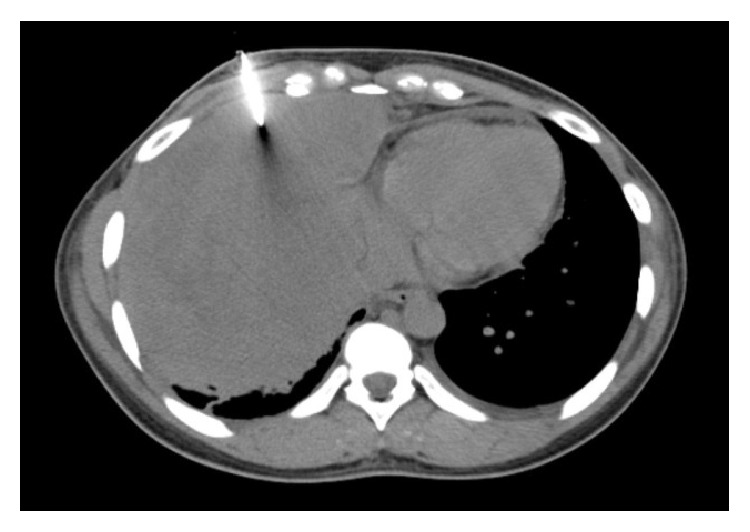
CT guided biopsy of mass.
